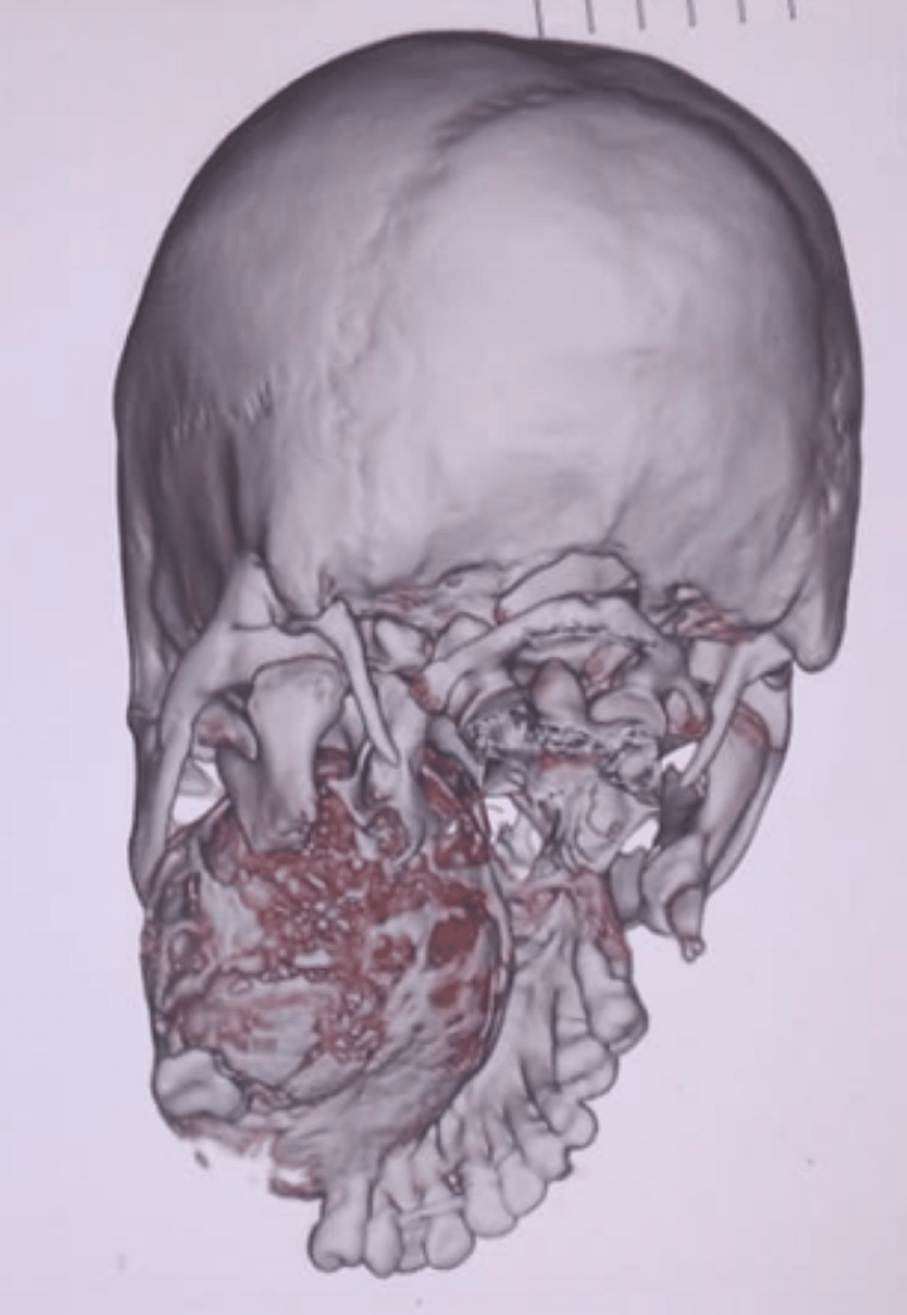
CT scan showing OF in the maxilla. The mandibular OF had been resected during the first surgery. CT: computed tomography; OF: ossifying fibroma
